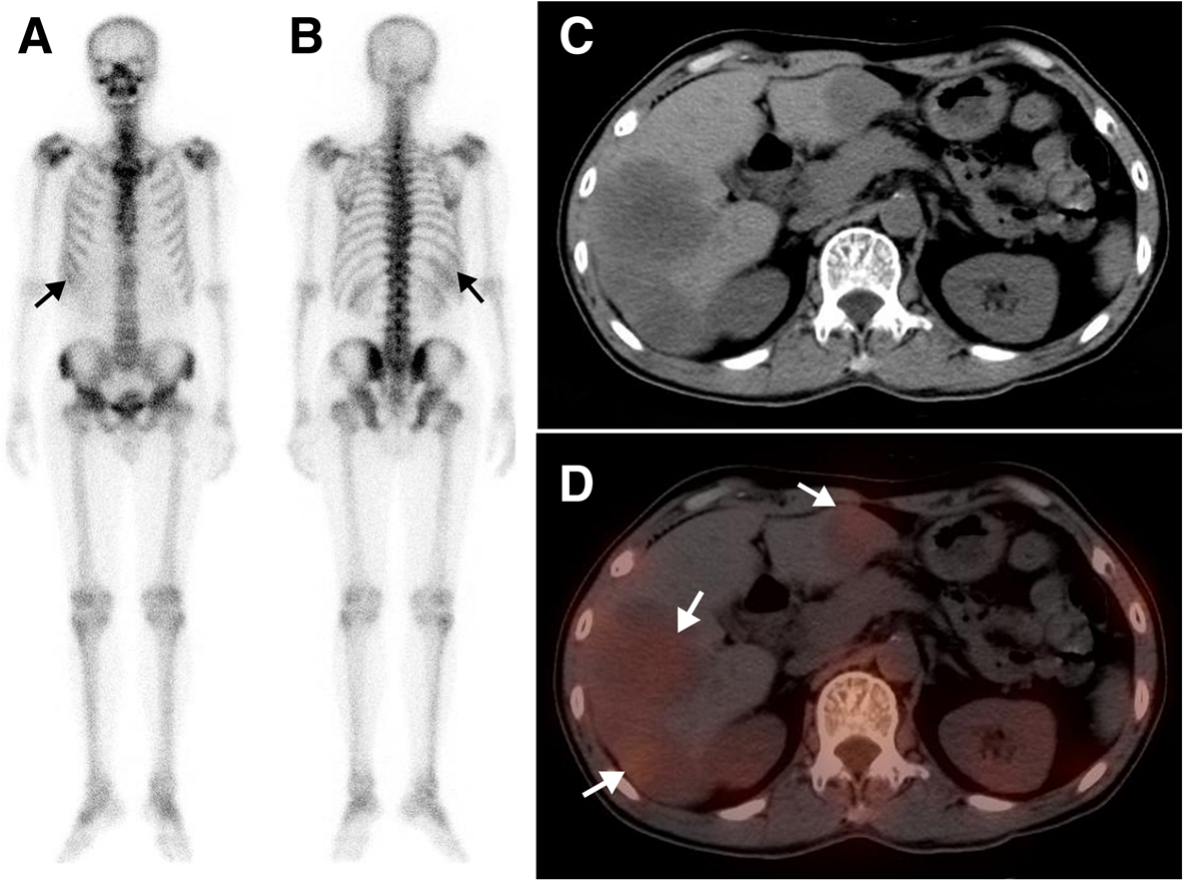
A 62-year-old man with rectal cancer after surgery and chemotherapy for 3 years, who was referred for suspected metastasis. The whole-body scan (a, anterior; b, posterior) demonstrated ^99m^Tc-MDP uptake in right upper abdomen (black arrow). Axial CT (c), and SPECT/CT images (d) showed increasing ^99m^Tc-MDP uptake corresponding to multiple liver metastases without calcification (white arrow)
